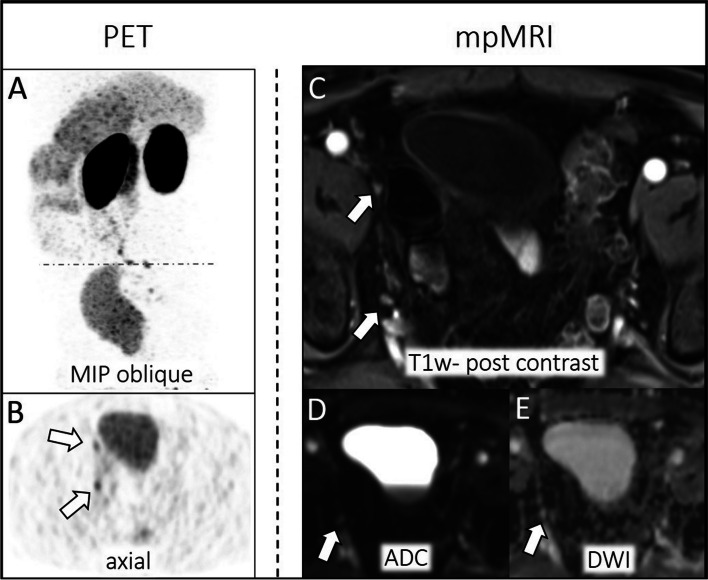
Pelvic lymph node recurrence of PCa parailiacal right. Lymph node metastases are detected by tracer accumulation (SUVmax: 9.3) at ^68^Ga-PSMA-11 PET (left A-B, white arrow: MIP oblique, axial dataset). Dashed line in MIP corona (A) indicates axial PET layer (B). Unsuspicious lymph node appearance at mpMRI (right C-E, white arrow: T1w post contrast, ADC, DWI)
